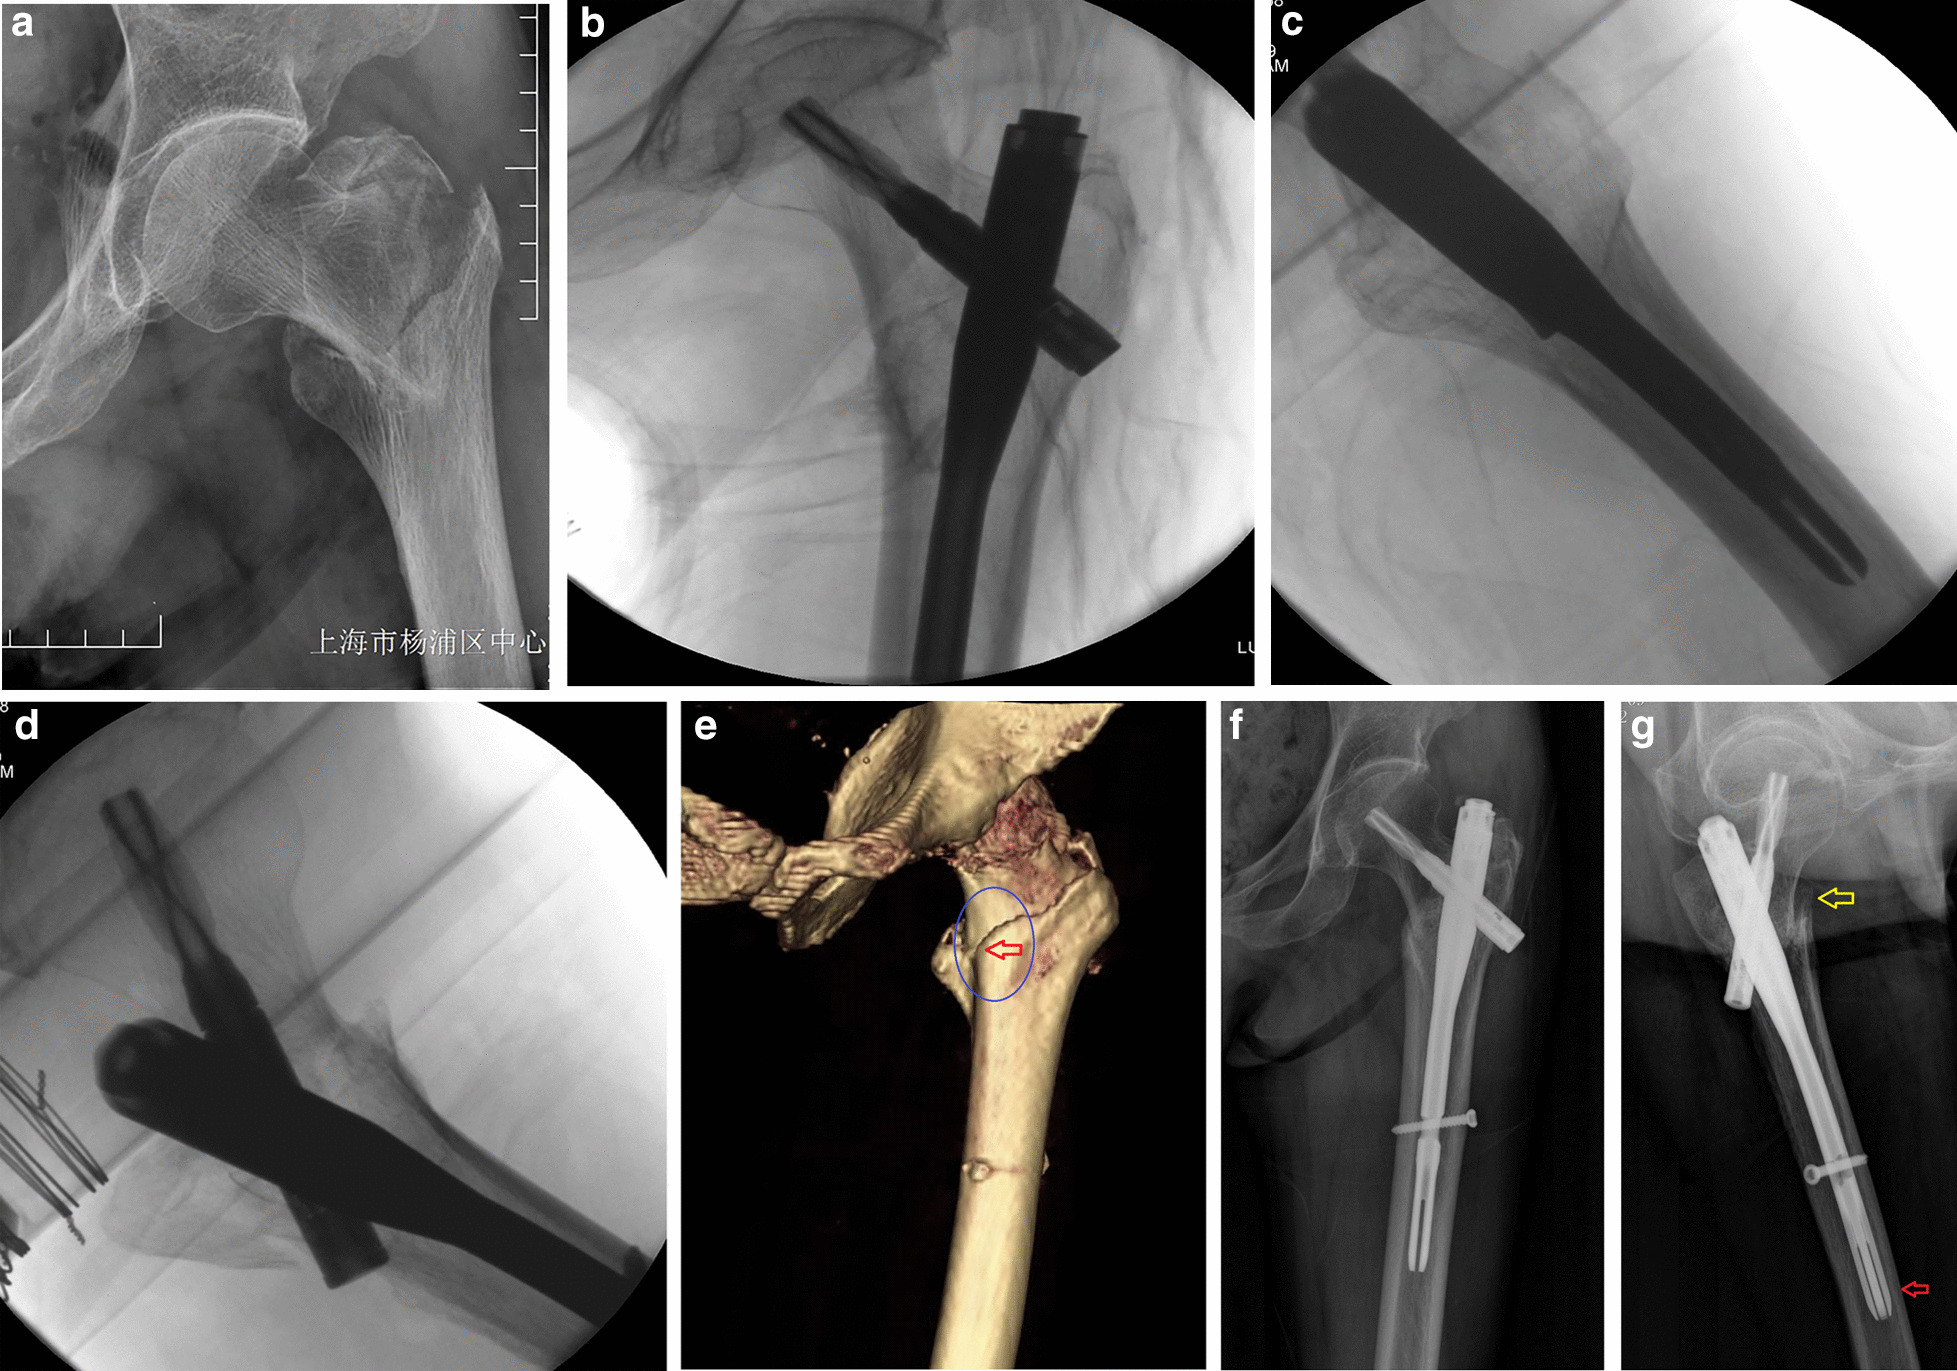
An 87-year-old woman with trochanteric femur fracture that treated by closed reduction and cephalomedullary nail fixation. a The fracture type was classified as 2018 AO/OTA 31A1.2. b–d Immediate postoperative fluoroscopy in AP, lateral, and anteromedial oblique views showed a good reduction quality, with smooth anteromedial cortex apposition (neutral relation). e Postoperative 3D CT image showed loss of cortical contact at anteromedial inferior corner. The proximal neck was sagged posteriorly into the distal medullary canal (the red arrow). f, g Follow-up X-ray in 6 months. AP view showed the backout of the helical blade by over-sliding, and lateral view showed loss of cortical contact of the anterior cortex with posterior sagging. The red arrow indicates anterior swing of the nail; the yellow arrow indicates negative anteromedial cortex relation, thus a sagittal pendulum-like movement was happened with lower filing ratio of distal nail/canal diameter
